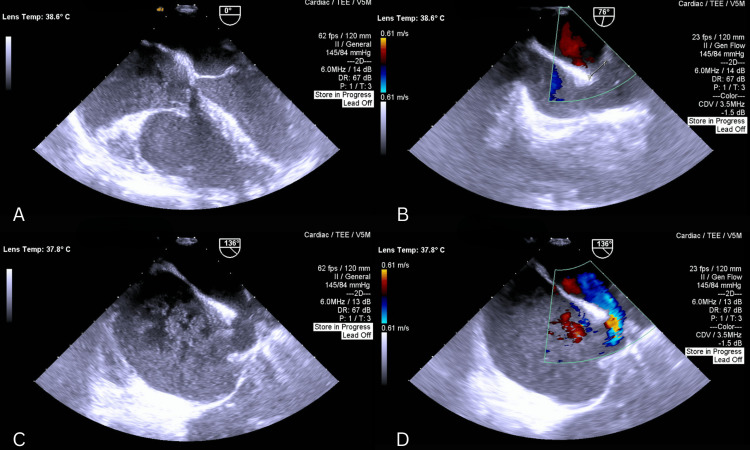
(A) Four-chamber view demonstrating enlarged RA and RV; TV is visualized; (B, C, D) Bicaval views showing the sinus venosus atrial septal defect (ASD) with left-to-right shunt on color Doppler (D)
